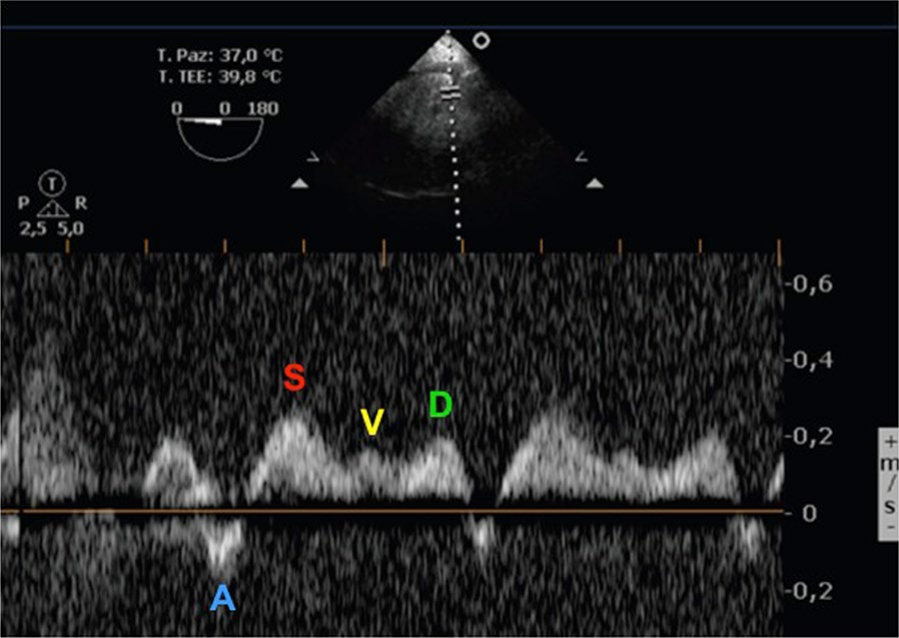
Normal triphasic hepatic Doppler waveform. The four waveforms constituting the normal spectral Doppler waveform are indicated: the A wave lies below the baseline (retrograde component); the S and D waves peak above the baseline and are caused by anterograde blood flow toward the right heart; and the V wave, a transitional wave, may peak just below or above the baseline
